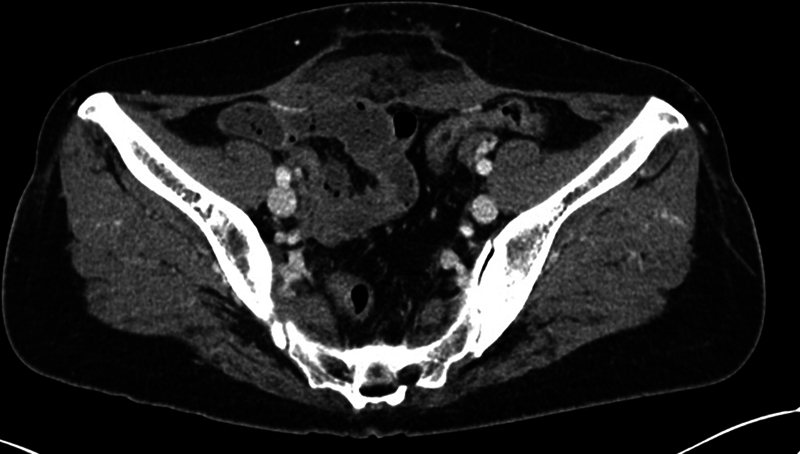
Axial contrast-enhanced CT image of case 1 showing abdominal wall hernia without overlying skin injury after HIFU therapy. Rectus abdominis muscle injury, fascia rupture, and subcutaneous fluid collection were noted 1 year after HIFU. CT, computerized tomography; HIFU, high-intensity focused ultrasound.
